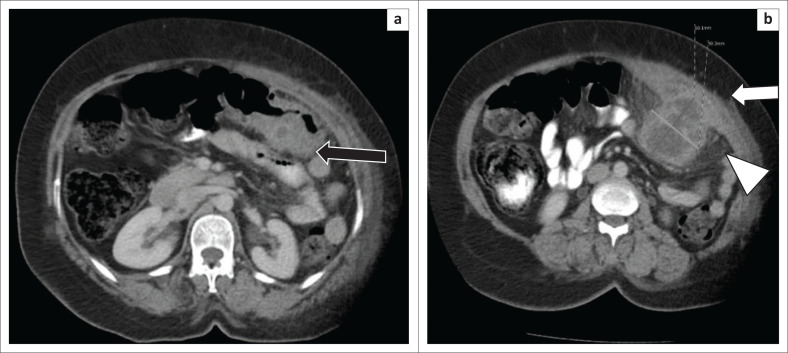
Selected axial computed tomography images demonstrated (a) an eccentric thickened wall of the distal transverse colon (black arrow) (b) with a focal, walled-off intra-abdominal fluid collection, extending from the wall of the transverse colon to the left anterior abdominal wall (white arrow). Invasion and thickening of the transversalis and rectus abdominis muscles was noted (white arrow). There was associated increased density and stranding in the pericolic fat and adjacent abdominal wall fat from the inflammation (arrowhead).
